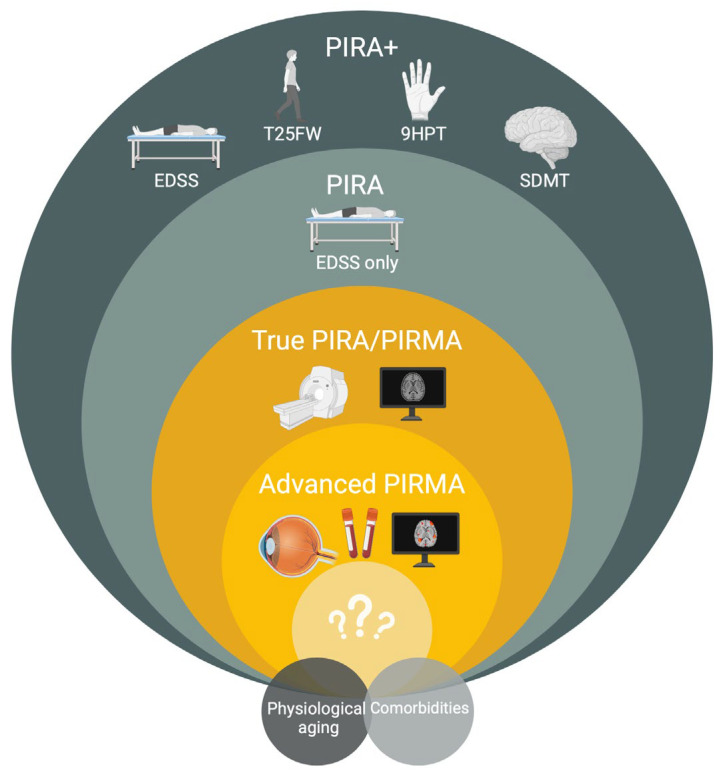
Peeling back the layers of PIRA. EDSS: Expanded Disability Status Scale. PIRA: progression independent of relapse activity. PIRMA: progression independent of relapse and magnetic resonance imaging activity. SDMT: Symbol Digit Modalities Test. T25FW: timed 25-foot walk test. 9HPT: 9-hole peg test.
